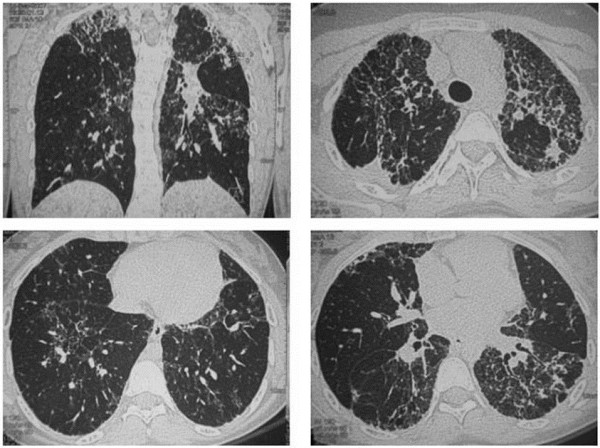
Patient A - chest CT at diagnosis. High-resolution CT scan demonstrates fibrotic architectural distortion with intra and inter-lobular thickening, associated with apical honey-combing. No ground glass opacity is present.
